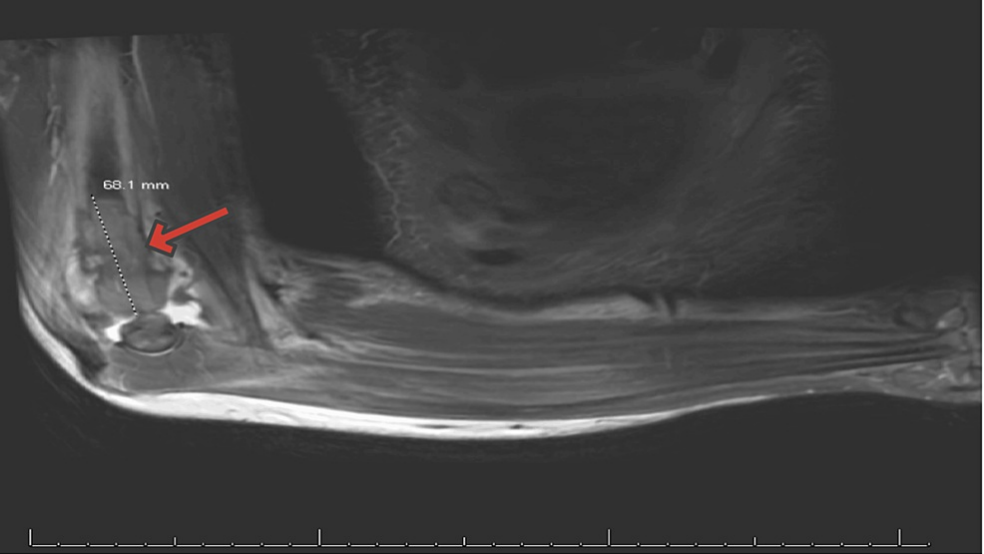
Right forearm MRI: expansile hypointense T1/T2 signal lesion at the distal humeral meta-diaphysis with avid enhancement measuring 6.8 × 5.3 × 5 cm (arrow), moderate elbow effusion is present, musculatures of the right forearm demonstrate preservation of signal intensity without fatty infiltration or denervation atrophy, and visualized joint spaces are preserved MRI: magnetic resonance imaging
